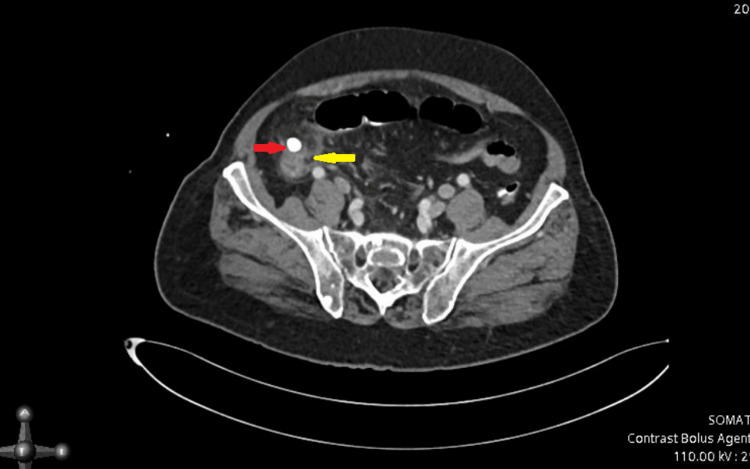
CT abdomen with contrast There is an ill-defined area of pericecal/right paracolic localized focal collection with fat stranding associated with adjacent bowel wall thickening involving the caecal base (yellow arrow). Approximately 10 x 9 mm hyperdense structure seen in the right iliac fossa likely appendicolith (red arrow). The appendix could not be seen separately.
